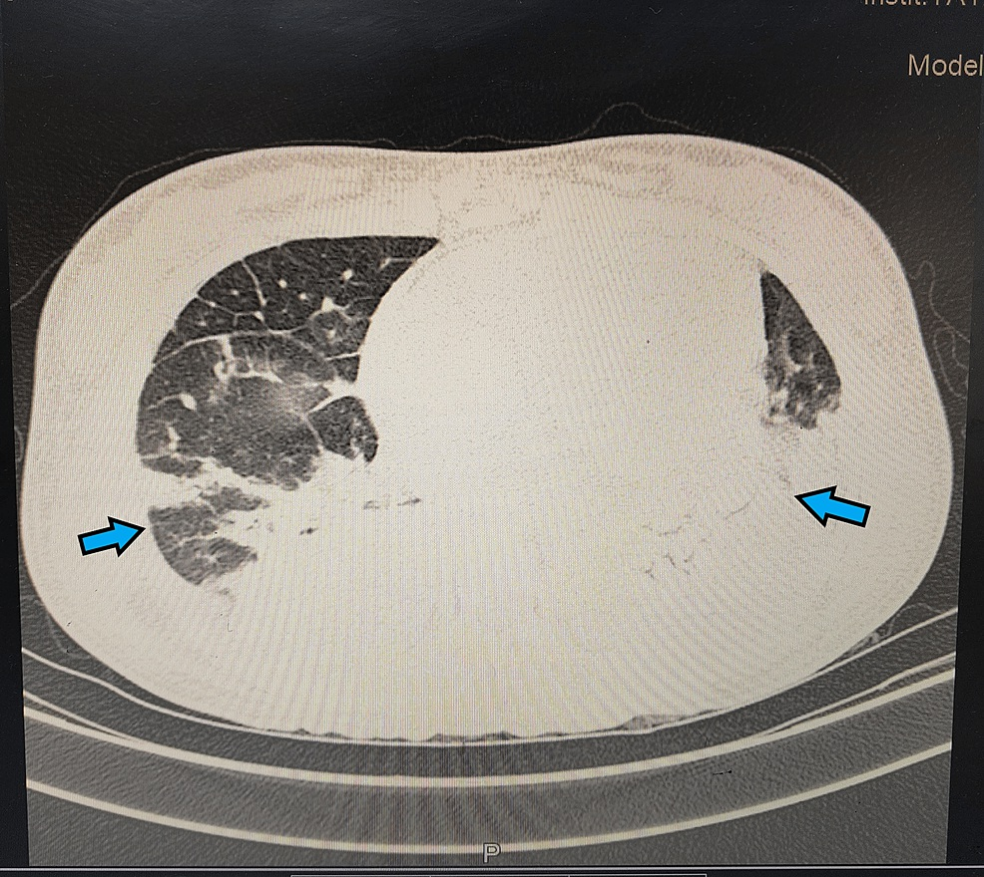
HRCT thorax showing diffuse ground glass opacities with interstitial thickening with a CT severity score of 12/25. HRCT: high-resolution computed tomography
